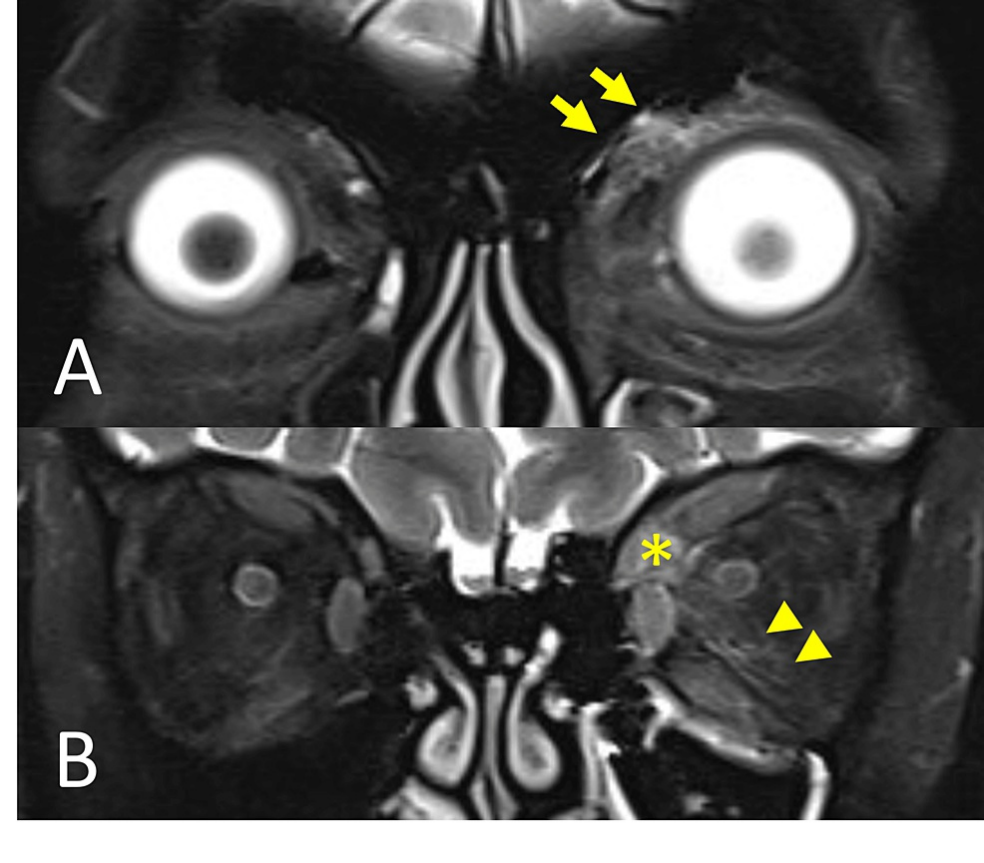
Coronal MRI orbit showed enhancing lesion over the left orbit. (A) Anterior orbital cut showed an enhancing ill-defined soft tissue lesion (arrow) over the extraconal superomedial periorbita compartment likely from the posterior orbit. (B) Posterior orbital cut showed an enhancing soft tissue lesion (asterisk) that occupied the superomedial compartment of the orbit while involved the superior rectus and medial rectus. Posterior two-thirds intraconal segment of the left optic nerve was surrounded by the extension of the lesion (arrowhead).
